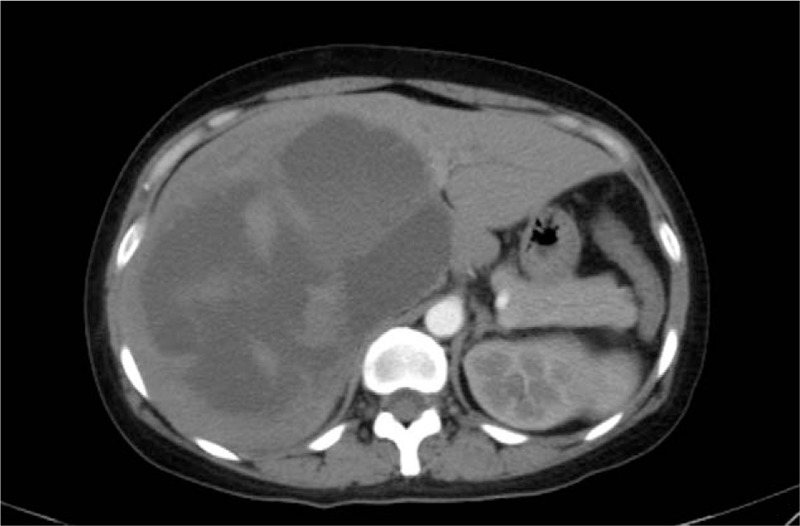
CT showed a huge cystic lesion sized 13.5 × 12 cm in the liver, and ascus and hemorrhage were observed in the lesion. CT = computed tomography.
